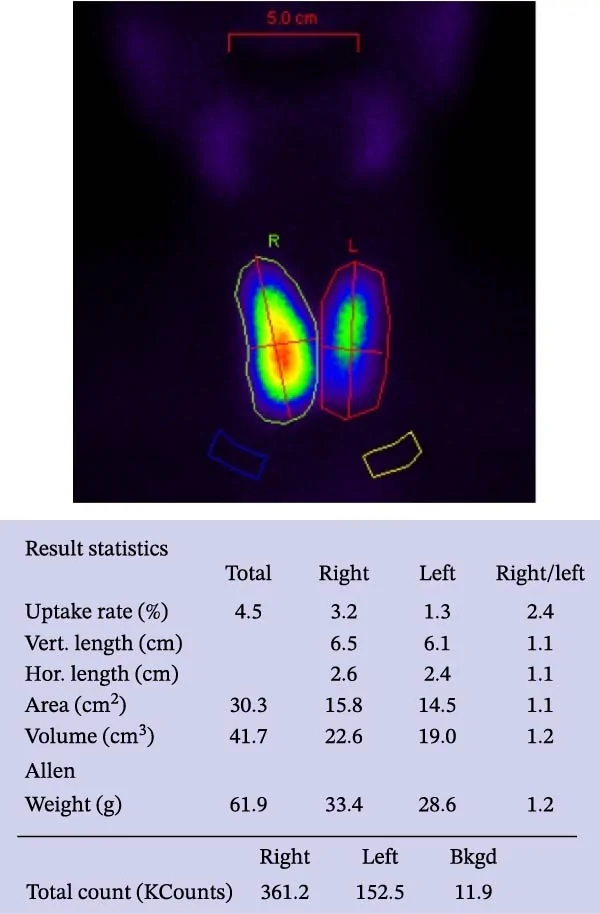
Technetium‐99 m thyroid scintigraphy demonstrating homogeneous uptake in the right lobe. The right and left lobes demonstrated an uptake of 3.2% and 1.3%, respectively.
